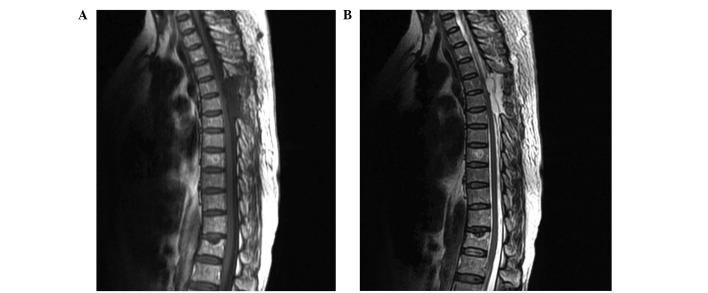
Postoperative (A) T1- and (B) T2-weighted magnetic resonance imaging (MRI) scans. The postoperative MRI scan, performed one week after surgery, demonstrates the complete resection of the mass.
